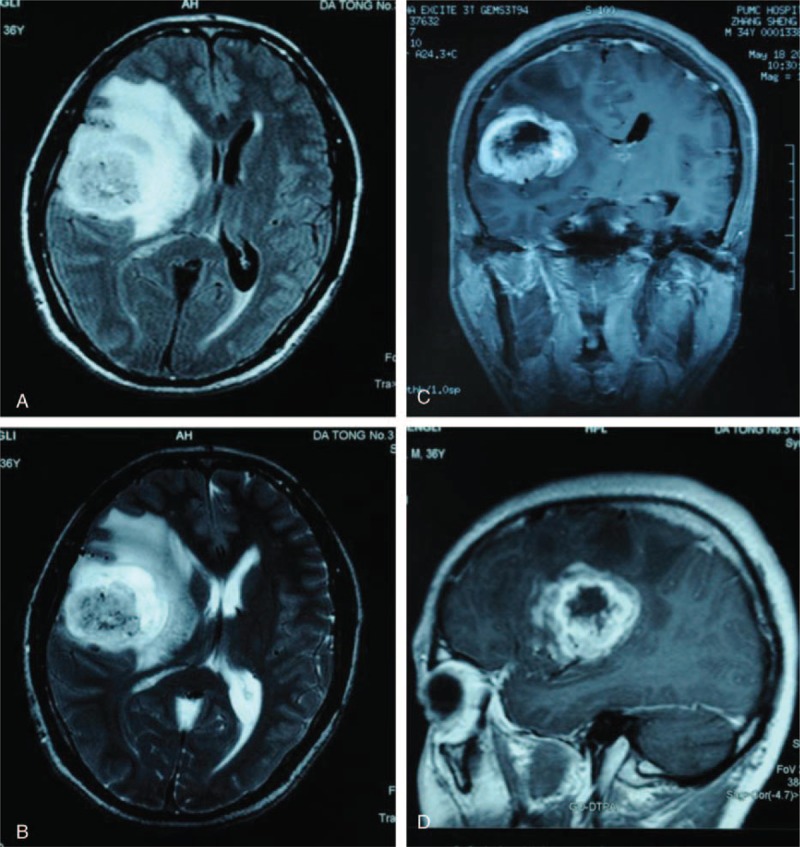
Radiological evaluation of the brain before surgery. T1-weighted axial gadolinium-enhanced magnetic resonance image demonstrates an enhancing tumor of the right frontal lobe (A, C, D). T2-weighted image demonstrates the same lesion as in the previous image, with notable tissue edema (B). This finding is consistent with a high-grade glioblastoma.
